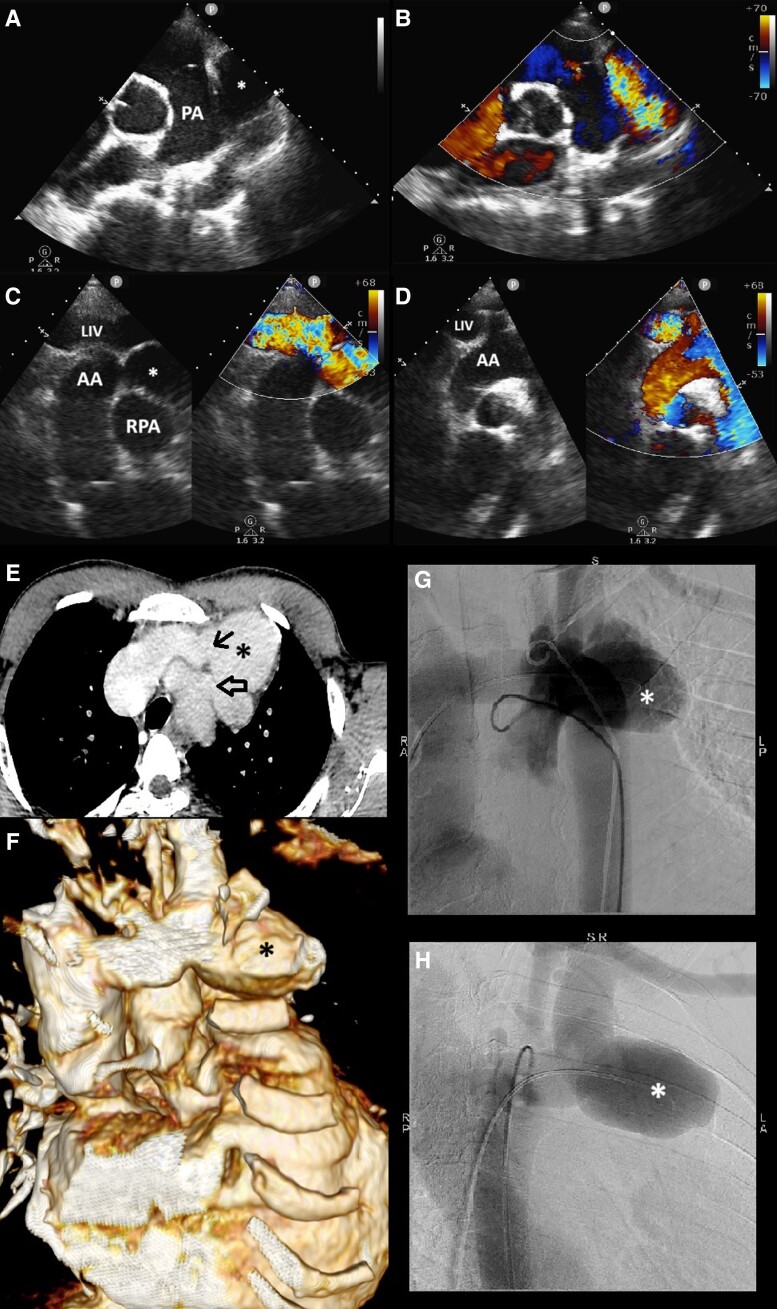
Transthoracic echocardiogram. (*A*) Parasternal short axis view showing a mass next to the pulmonary artery. (*B*) Same view with colour Doppler activated showing intense aliasing. (*C*) and (*D*) Suprasternal view showing the fistula with the left innominate vein. Computed tomography angiography. (*E*) Superior mediastinal axial view showing an arteriovenous communication between the innominate trunk (thin arrow) and the aortic arch (thick arrow). (*F*) 3D reconstruction. Aortic angiography. (*G*) Left anterior oblique view showing a large pseudoaneurysmal structure with a shunt between the aortic arch and the left innominate vein. (*H*) Right anterior oblique view. Star: pseudoaneurysmal vascular structure. AA: aortic arch; LIV: left innominate vein; PA: pulmonary artery; RPA: right pulmonary artery.
